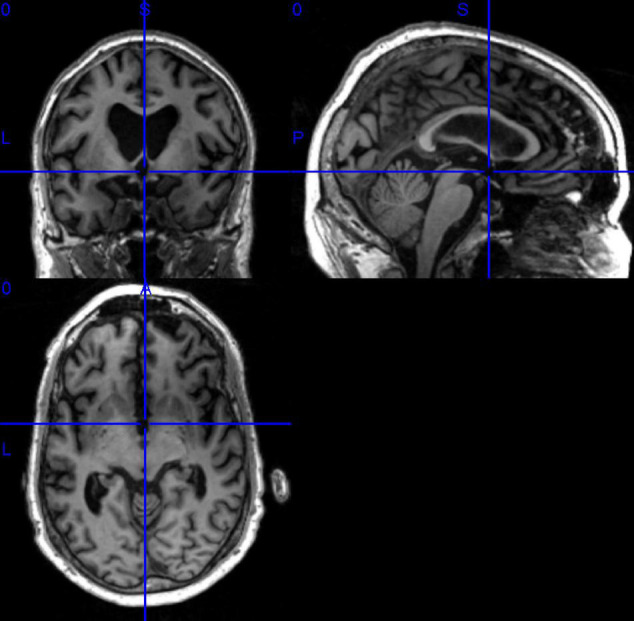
Example of 3D MRI image.
